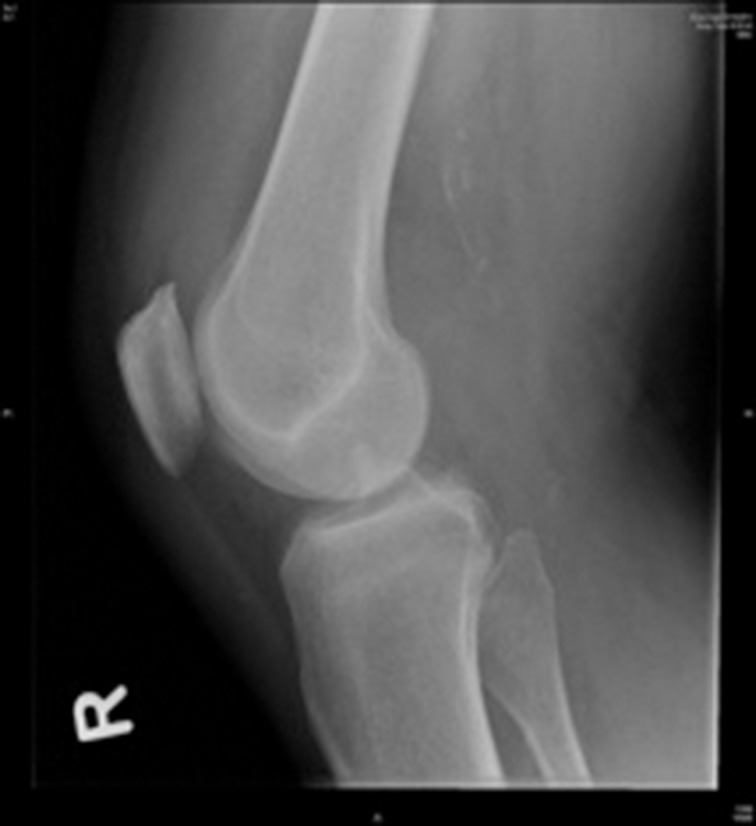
Anteroposterior and lateral knee x-ray taken at initial presentation, demonstrating cyamella behind lateral femoral condyle
